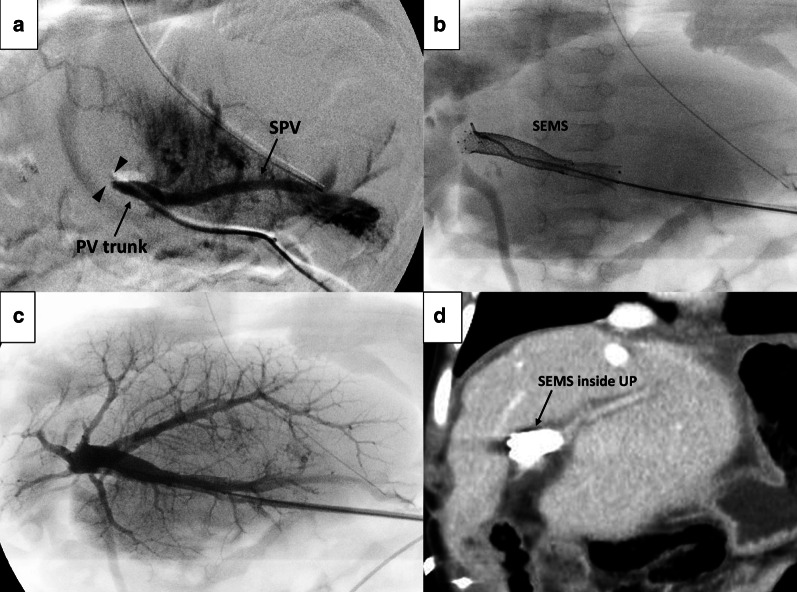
Intraoperative portal venograms (a–c) and a postoperative computed tomography (CT) image (d) (Case 2). a A portal venogram showing a complete obstruction of the PV anastomosis (arrowheads) and a regurgitating flow to the splenic vein (SPV). b Two overlapping SEMSs were implemented to dilate the portal vein anastomosis. c The portal venogram after the successful stenting. The PV flow was completely restored from the PV trunk to the intrahepatic portal vein branches. d A CT image at 3 postoperative months showing patent SEMS and sufficient portal venous flow in the liver graft
